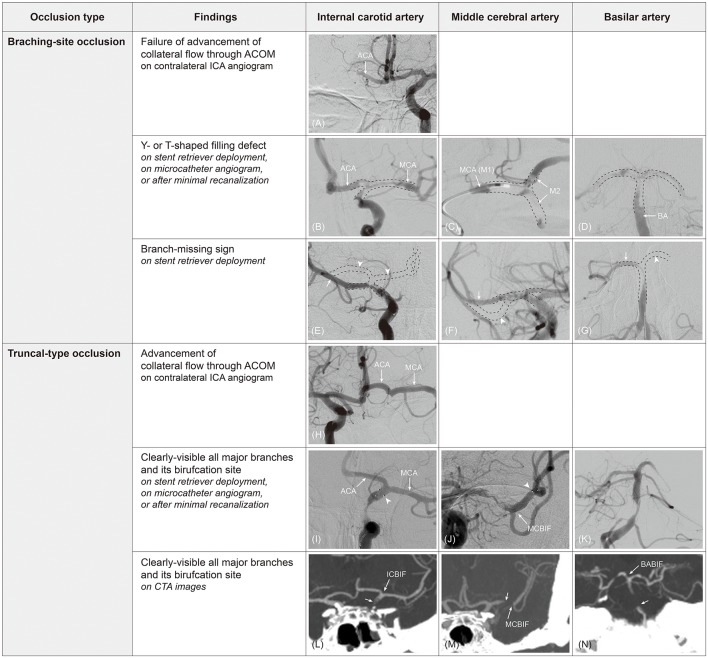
Determination of occlusion type by digital subtraction angiography (DSA) and computed tomography angiography (CTA). On contralateral internal carotid artery (ICA) angiogram, collateral flow cannot advance to ipsilateral middle cerebral artery (MCA) through anterior communicating artery (ACOM) because ipsilateral ICA bifurcation (ICBIF) site is involved in branching-site occlusion (A). On the contrary, collateral flow can progress to ipsilateral MCA in truncal-type occlusion (H). In branching-site occlusion, Y- or T-shaped filling defect (clot) involving arterial bifurcation site can be observed on stent retriever deployment (B), on microcatheter angiogram (C), or after minimal recanalization (D). In addition, owing to the involvement of arterial bifurcation site, only one branch that stent retriever is deployed to can be seen on post-deployment angiogram in branching-site occlusion [arrow in (E–G)], while another branch is not seen [missing branch sign; arrowhead in (E–G)]. In truncal-type occlusion, all major branches and its bifurcation site can be clearly observed by stent retriever deployment [(I,J); arrowhead, distal markers of stent retriever], on microcatheter angiogram beyond occlusion, or after minimal recanalization (K). Those can also be observed on CTA images [(L–N); arrow, original occlusion point]. ACA, anterior cerebral artery; M2, superior and inferior divisions of middle cerebral artery; MCBIF, middle cerebral artery bifurcation site; BABIF, basilar artery bifurcation site.
